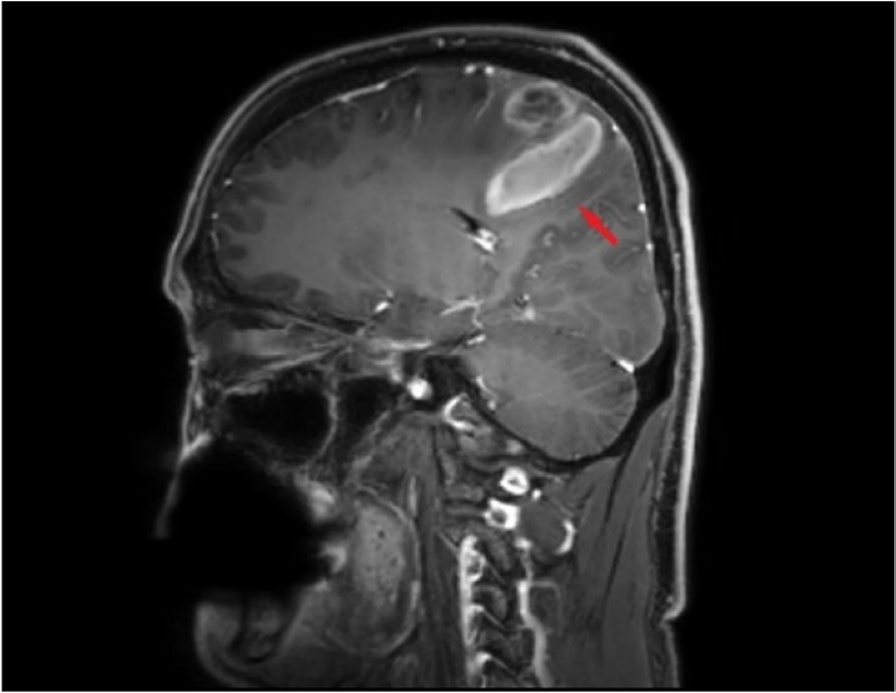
Enhanced magnetic resonance imaging shows meningeal and peripheral enhancement, but no significant enhancement in the hemorrhage area
